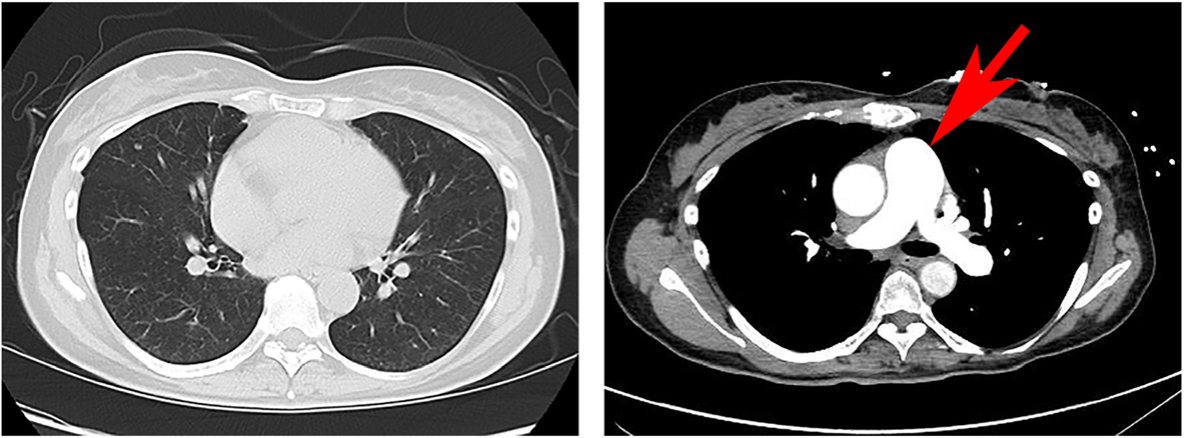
Computed tomographic (CT) images obtained after the patient’s arrival and admission to the hospital. Left panel: thoracic CT image obtained after the patient’s arrival to the hospital shows no abnormal lesion. Right panel: thoracic contrast-enhanced CT obtained after the patient’s admission to the hospital shows no massive pulmonary thromboembolism or tumor emboli. The red arrow indicates aorta enlargement
